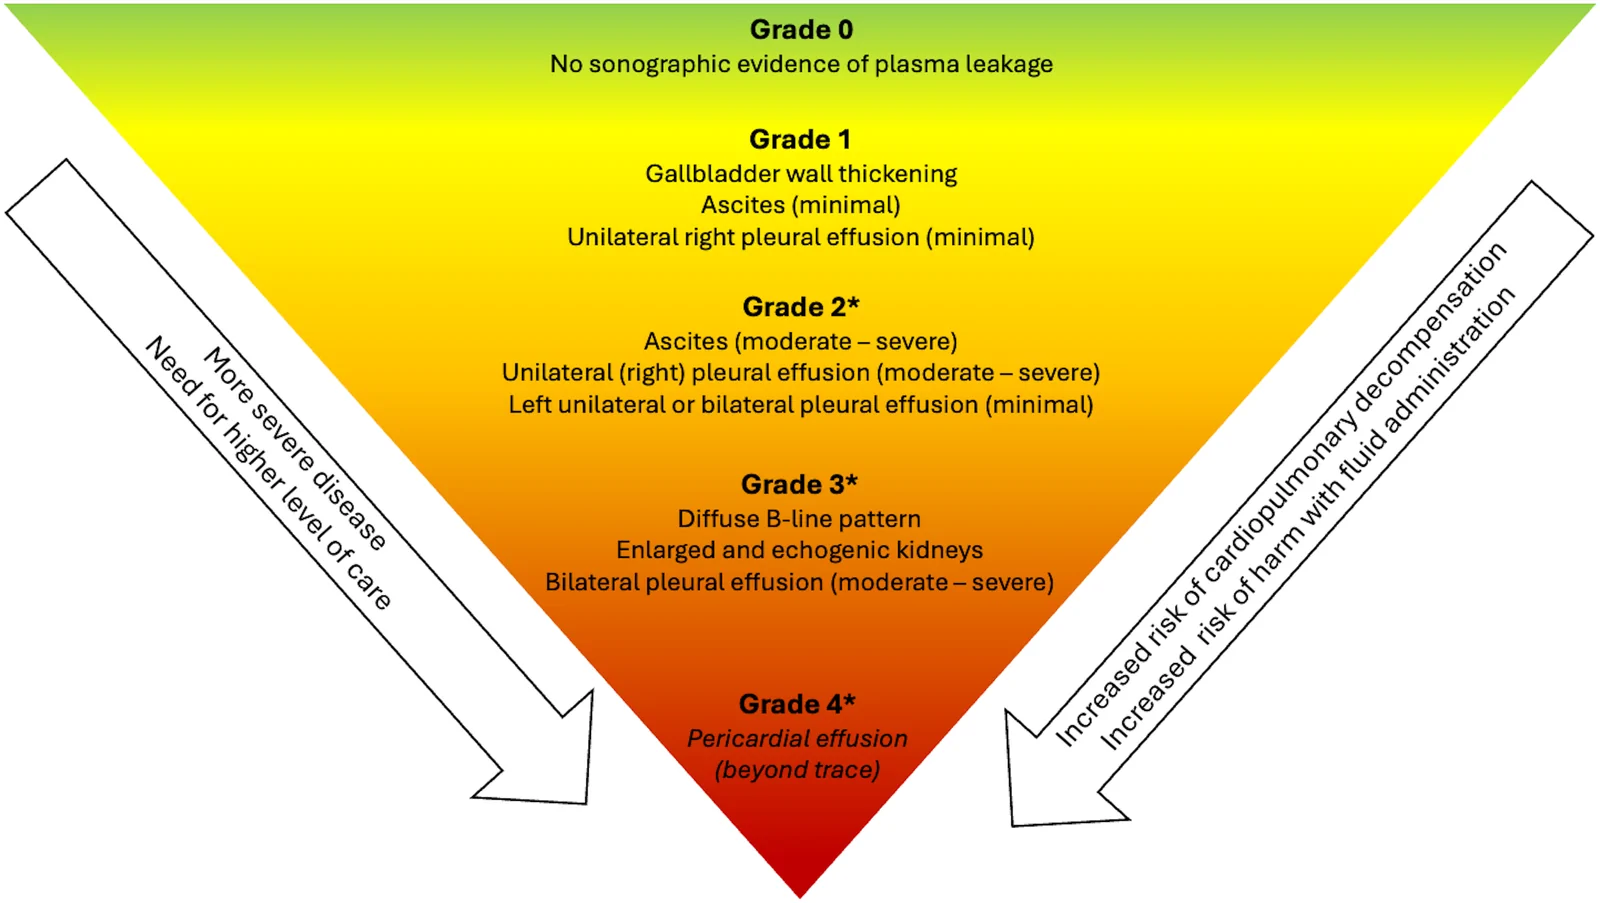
Ultrasound-based assessment of Plasma leakage Severity (UPS) for patients with viral infections associated with hemorrhagic fevers and with stable hemodynamics and respiratory status. *Each higher grade may include any findings from lower grades as well as any finding for that grade. For example, Grade 3 may include gallbladder wall thickening and ascites from lower grades along with any of the Grade 3 criteria: diffuse B-lines or enlarged and echogenic kidneys or bilateral pleural effusions. Source: This is an original image created by the authors; please cite this current manuscript.
